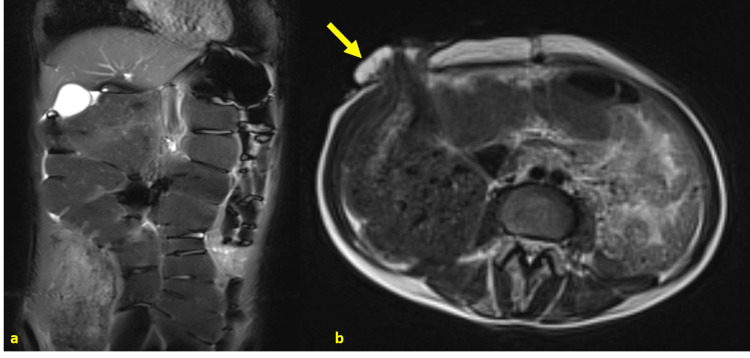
T2-weighted MRI (coronal and axial views) showing diffuse colonic distension. Note the right colostomy opening a: coronal view
